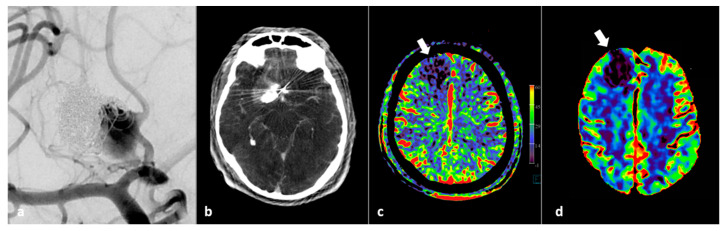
FD CT PBV imaging in a 46-year-old patient with thrombo-embolic and hemorrhagic complications during flow diverter placement. (a) AP view DSA image pre-intervention shows a significant neck remnant after previously coiled pericallosal aneurysm. (b) FD CT axial MIP reformation after occurrence of complications shows local hematoma and contrast. (c) Post PVO FD CT imaging shows right frontal residual wedge shaped perfusion deficit (white arrow). (d) Follow-up conventional MD CT CBV perfusion confirms local perfusion deficit.
